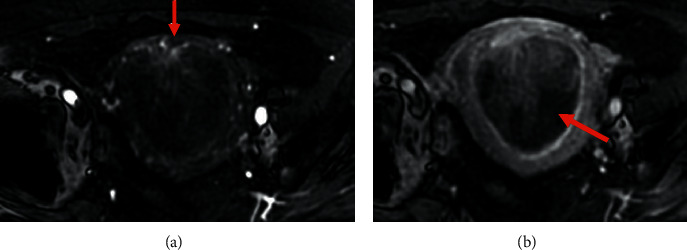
Pelvic MRI of endometrial stromal sarcoma: (a, b) axial DCE-MRI showing tumoral vessels in the lesion at arterial phase and disruption of arcuate arteries subendometrial ring (arrow) (a) and percentage of necrosis at venous phase (arrow) (b).
